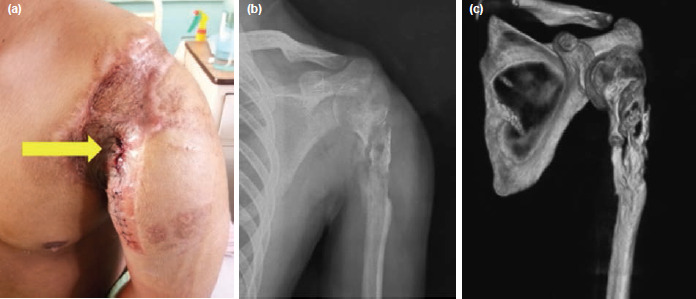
(a) Two months after the initial surgery, he presented with persistent sinus discharge (yellow arrow) at the anterolateral aspect of his left shoulder. (b) Plain radiograph of the left humerus showed sequestrum located at the proximal part of the left humerus. (c) Computed tomography of the left humerus provided a detailed delineation of the sequestrum, thus assisting in the planning of bony resection to remove the sequestrum.
